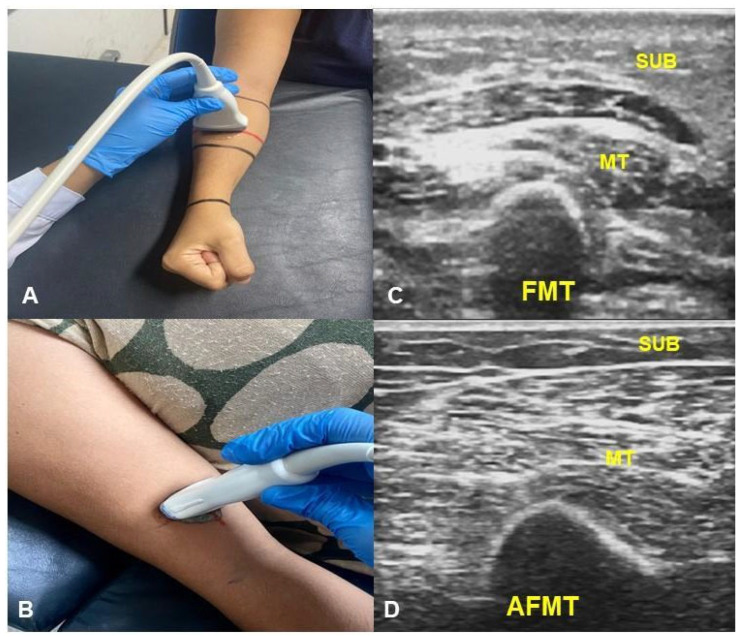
(A) Patient and transducer positioning for ultrasound imaging of forearm flexors (FMT); (B) Ultrasound image of FMT; (C) Patient and transducer positioning for ultrasound imaging of arm flexors (AFMT); (D) ultrasound image of AFMT. SUB: subcutaneous tissue; MT: muscle thickness. Source: The authors (2005).
